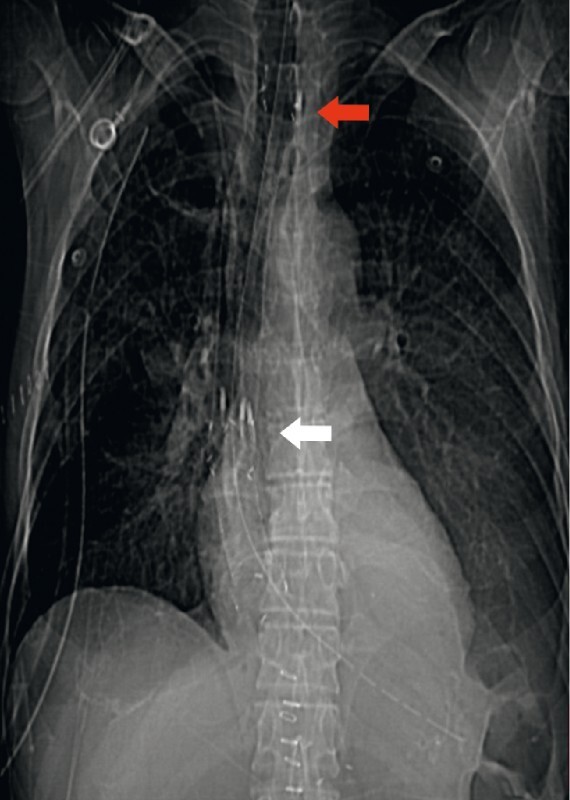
Radiographic image showing the two correctly located stents, with the upper stent fixed at its cranial end in the cervical esophagus (red arrow) and the two stents secured together with clips (white arrow).
